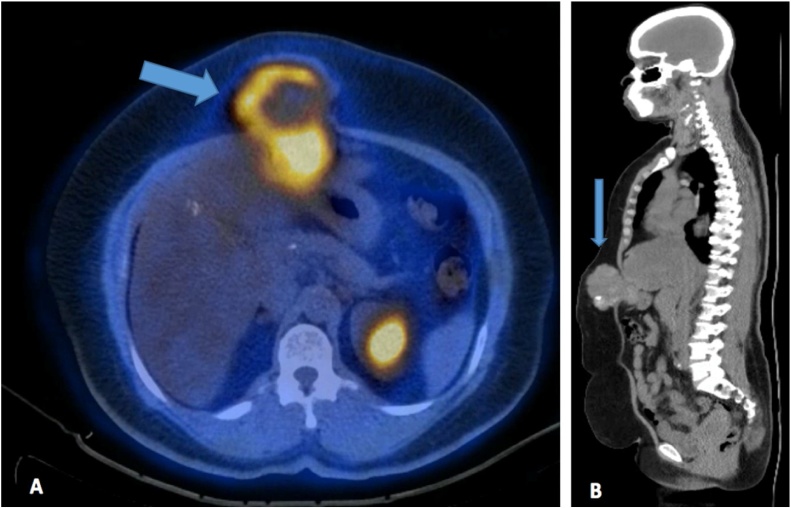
A: Positron emission tomography-CT of chest, abdomen, and pelvis revealing post-surgical changes in the liver with no focal uptake suggestive of local recurrence. Fatty changes are noted. A soft tissue lesion with areas of coarse calcification is seen in the anterior abdominal wall on the right, next to the midline; it is in close proximity to the site of the previous surgical incision, and measures 8.3 × 6.9 cm, with a maximum standardized uptake value of 6.5. B: Sagittal view of the same image.
